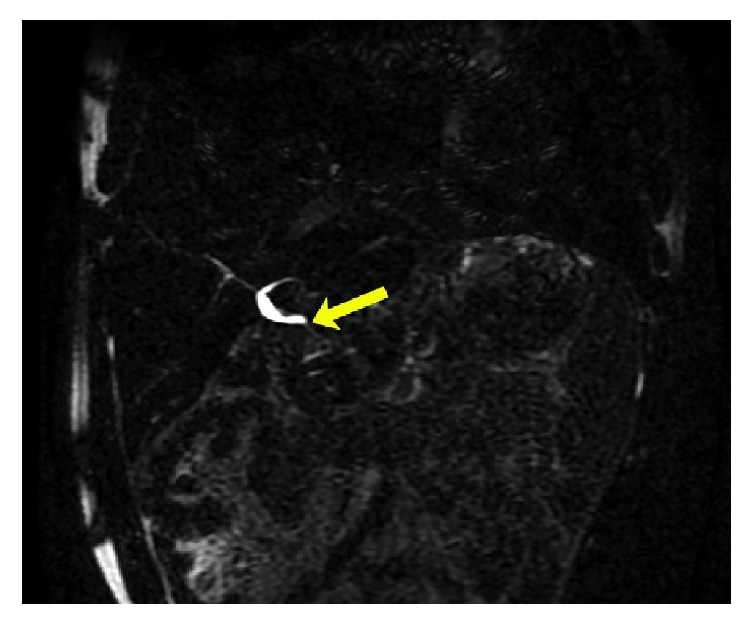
MRI/MRCP showing anastomotic biliary stricture, common hepatic ductal dilatation, and subtle curvilinear filling defect within the common hepatic duct.
